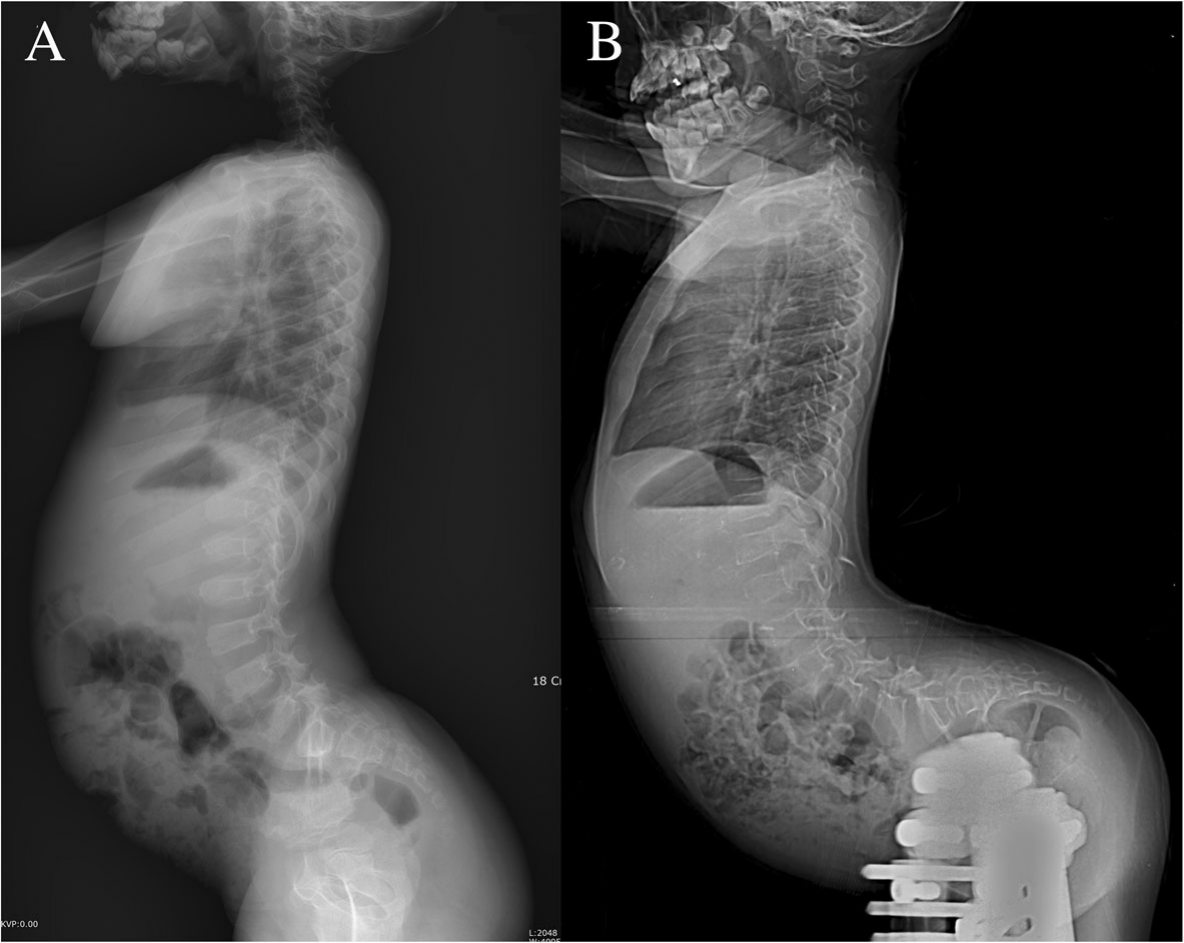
Radiographs of a 10-year-old boy with achondroplasia exhibiting sustained hip flexion contracture after femoral lengthening. a Initial radiograph of the patient. The patient underwent simultaneous bilateral femoral lengthening to gain 11 cm (lengthening percentage of 42.9%). b Postoperative radiograph showing an aggravation of the horizontal sacrum with lumbosacral hyperlordosis
